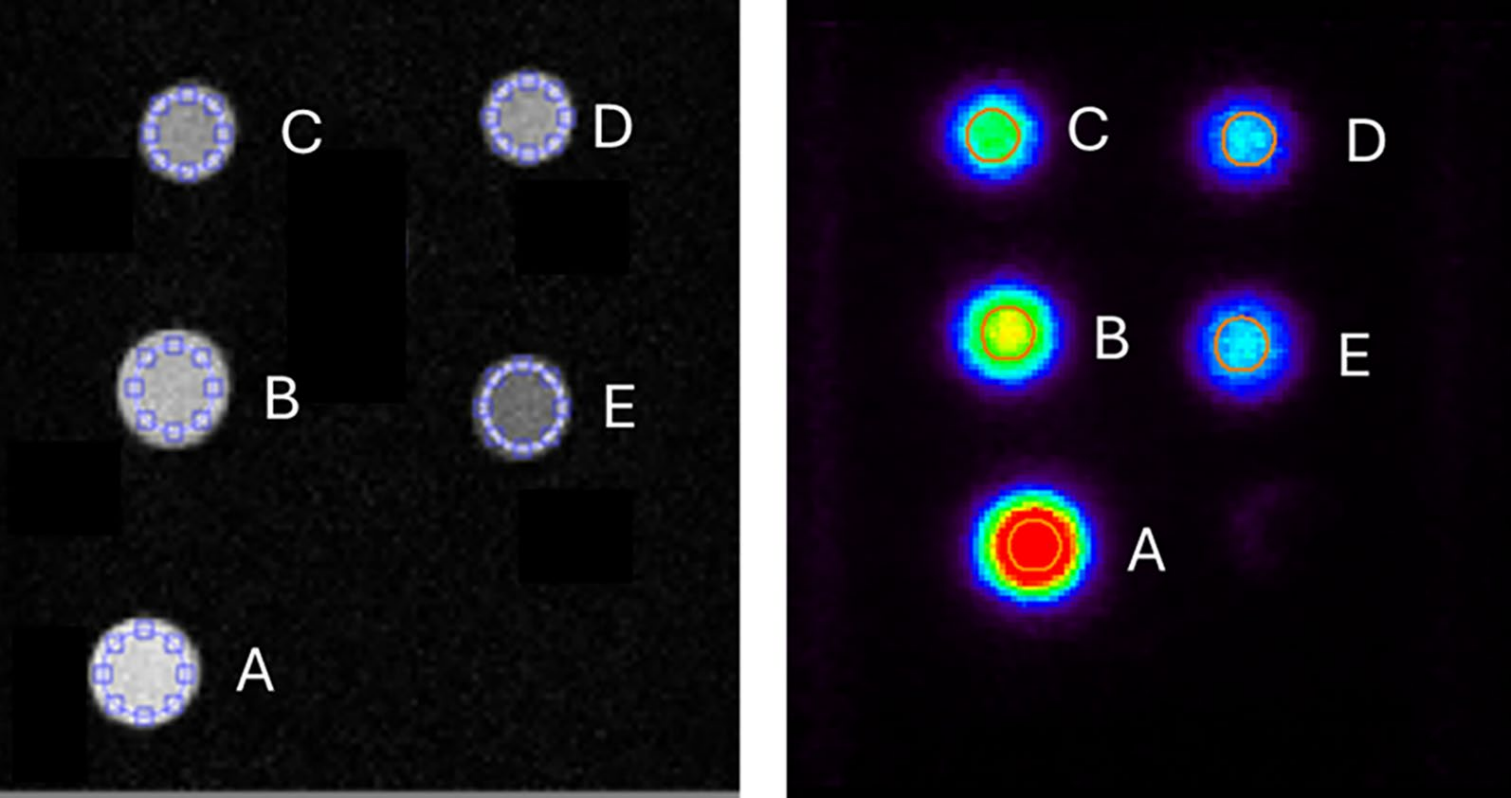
The MRI (left side) and PET scans (right side) of the dual imaging agent in five different concentrations and pH (A-E)
